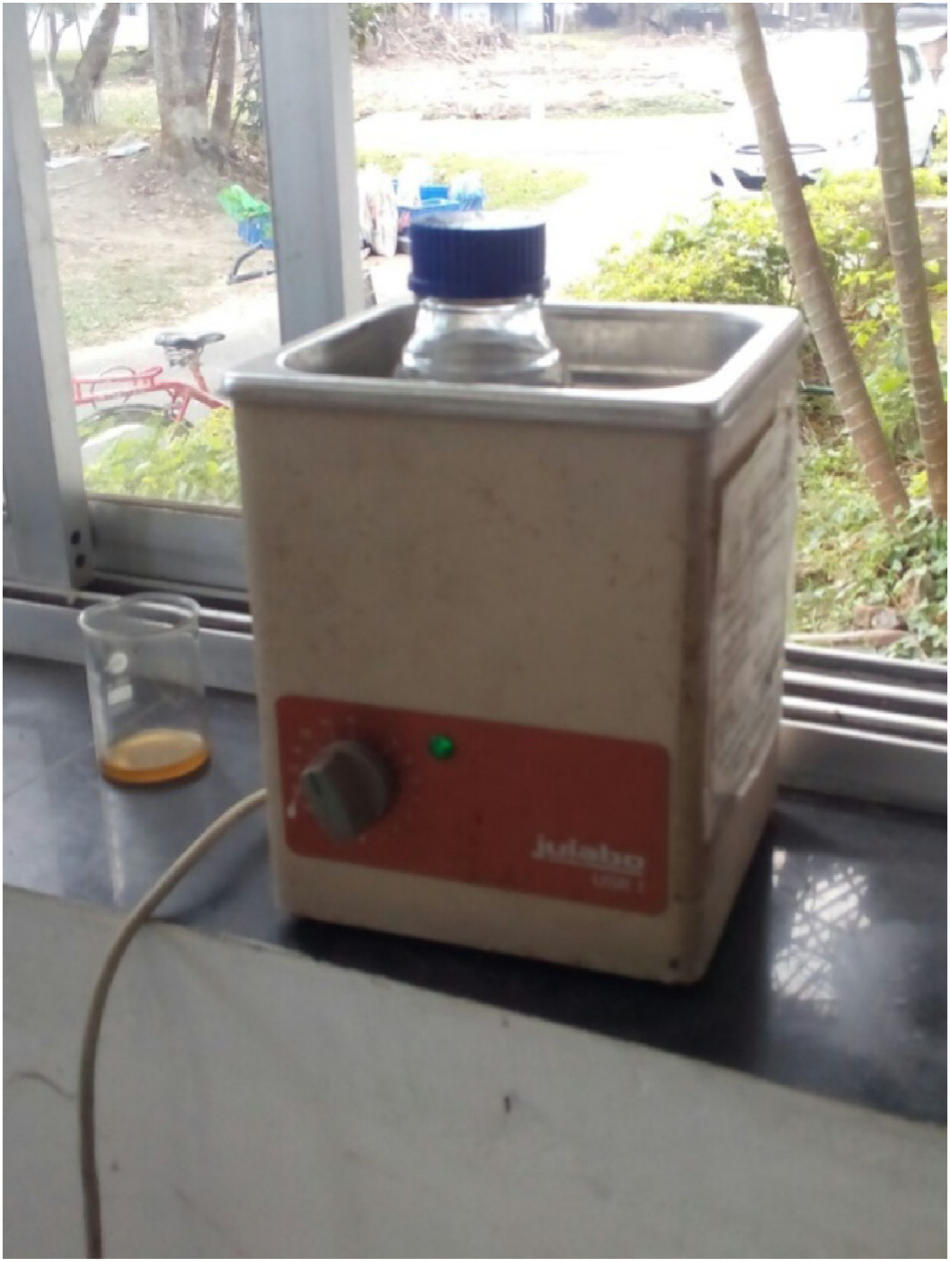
Preparation of stock solution in the ultrasonic bath.
